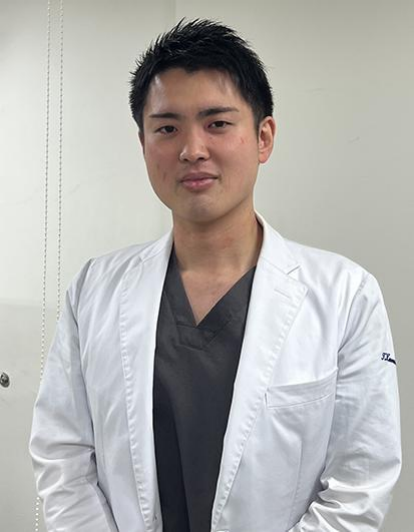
A cardiologist specializing in cardiovascular intervention, structural heart disease, and echocardiography. Actively involved in the care of congenital heart disease patients by applying expertise in these specialized fields.
